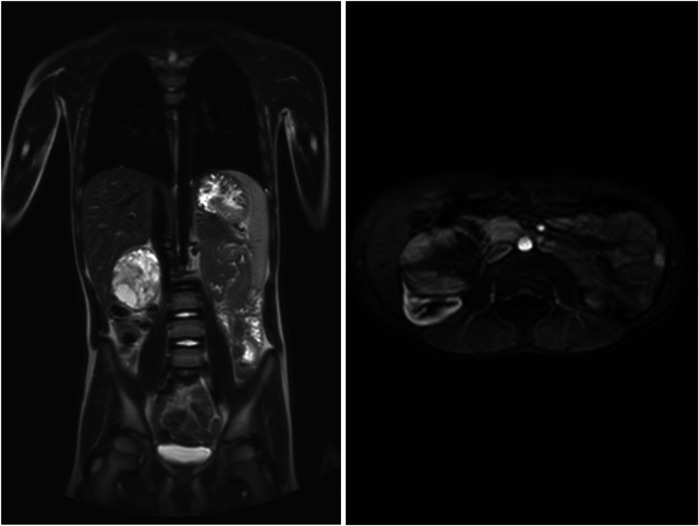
Metanephric adenoma in magnetic resonance image.
